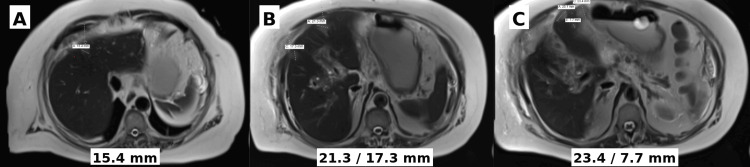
Axial T2 Weighted MRI Abdomen Axial T2-weighted MRI of the abdomen showing multiple hyperintense lesions concerning for liver metastases. Arrows and measurements were added in the imaging software by the radiologist and cannot be modified or removed; larger font is included in each corresponding image. (A) A 1.5 cm lesion in segment 4A of the liver; (B) a 1.7 cm lesion in segment 8 of the liver and a 2.1 cm lesion in segment 2/3 of the liver; (C) a cluster of three lesions in liver segment 3 measuring 2.3 cm, 2.3 cm, and 0.8 cm.
